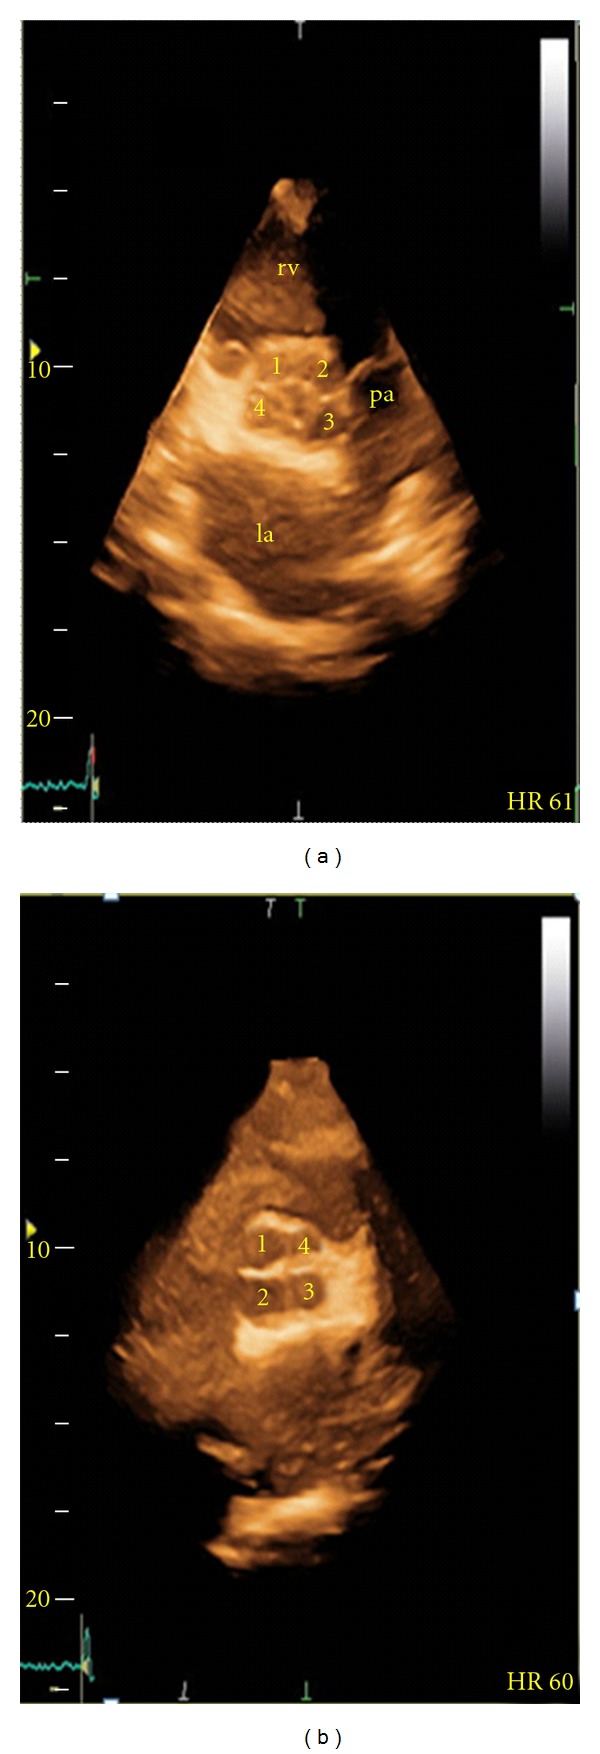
3-dimensional transthoracic echocardiography images. Short axis view during early systole (a) and diastole (b). pa: pulmonary artery, la: left atrium, and rv: right ventricule.
